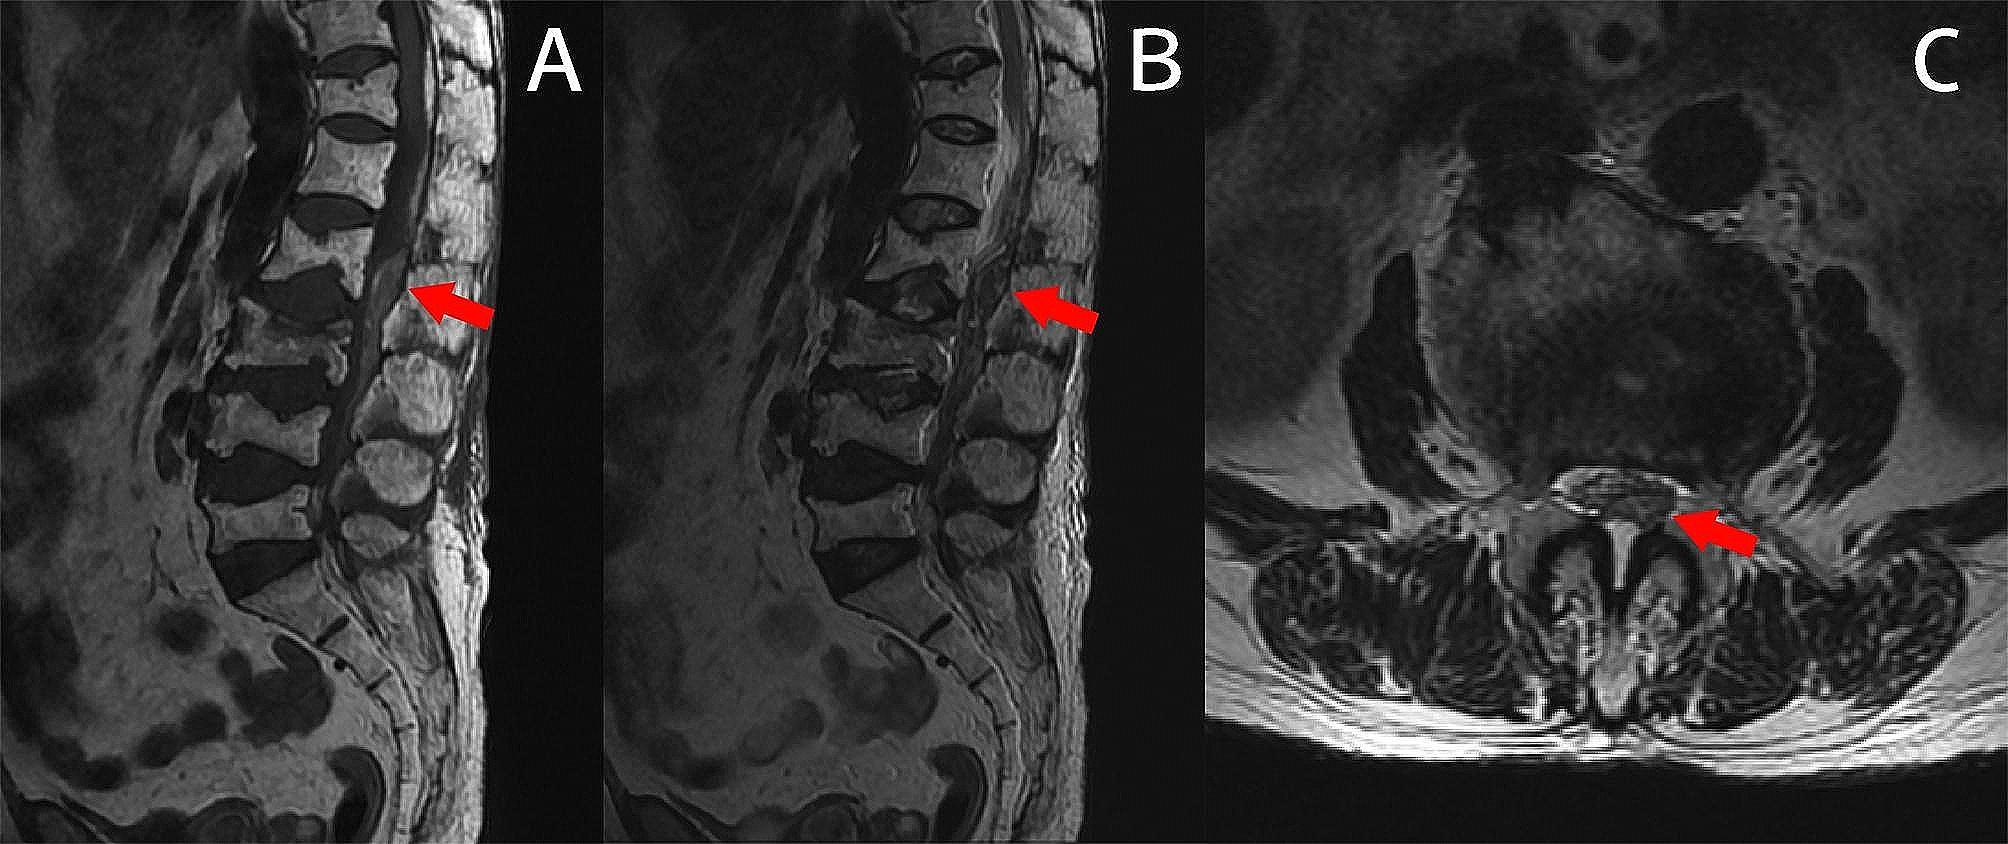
Follow-up MRI four days later revealed that the SEH (red arrow) had decreased in size (A-C). SEH: spinal epidural hematoma. MRI: magnetic resonance imaging. WI: weighted imaging
